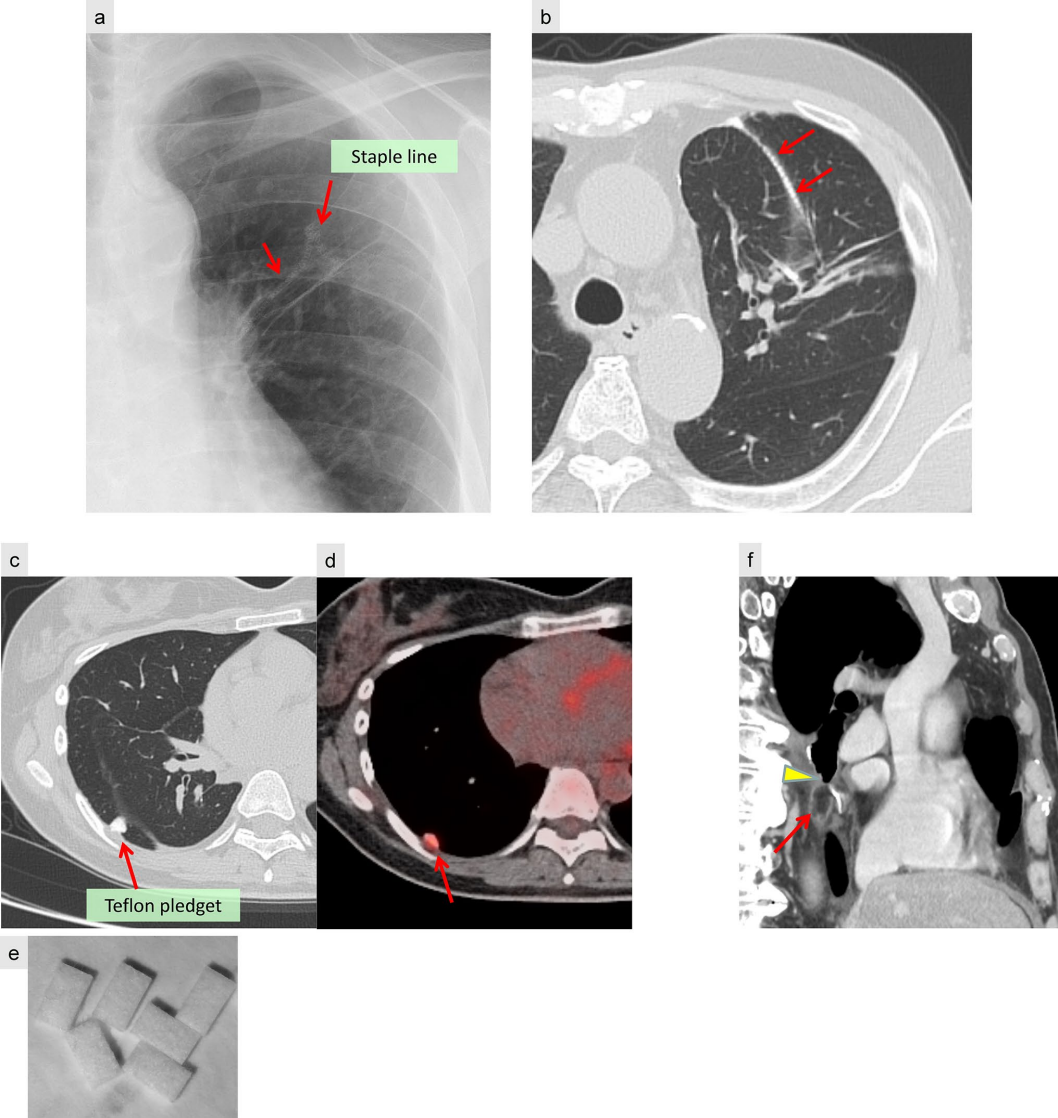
Surgical materials and fat pad. a, b After left S3 segmentectomy, CXR (a) and CT (b) reveal a linear high-attenuation structure corresponding to the staple line (arrow), indicative of suture materials. c, d, e Following right lower lobectomy, the Teflon pledget appears as a polygonal nodule with high attenuation on CT lung window settings (c), positioned in contact with the pleura and interlobar pleura. FDG-PET imaging demonstrates associated radiotracer accumulation (d). A photograph of the Teflon pledget is also provided (e). After right lower lobectomy, the bronchial stump is covered by free pericardial fat tissue (f). The sagittal CT image reveals a soft-tissue mass with fat attenuation (arrow) at the distal end of the bronchial stump (arrowhead)
